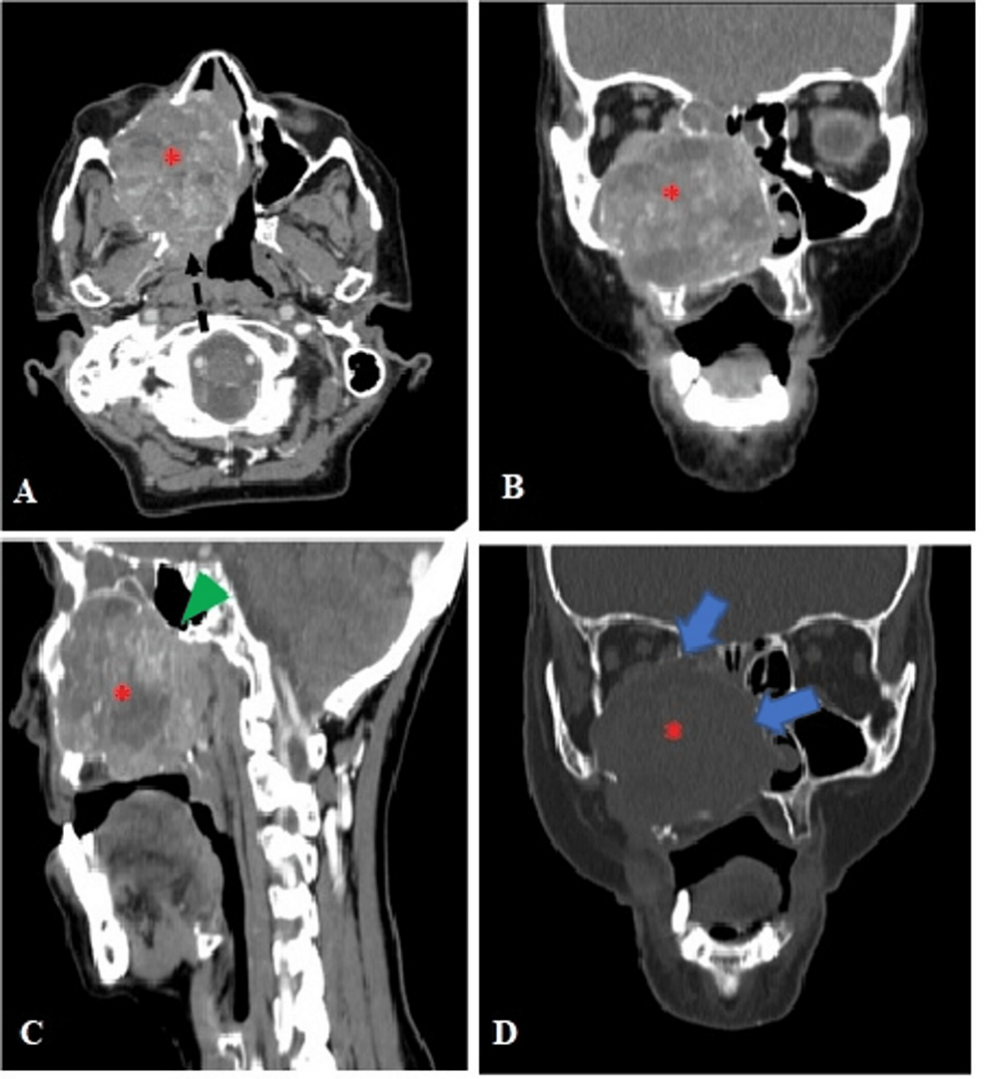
A 66-year-old patient with a mass in the right maxilla. Contrast CT of the head and neck showing an expansile lesion with heterogeneous contrast enhancement in the right maxillary sinus (asterisk) (A-D), infiltration of the pterygopalatine fossa (dashed arrow) (A), and the sphenopalatine foramen (arrowhead) (C). The bone window CT shows bone remodeling (arrow) (D).
